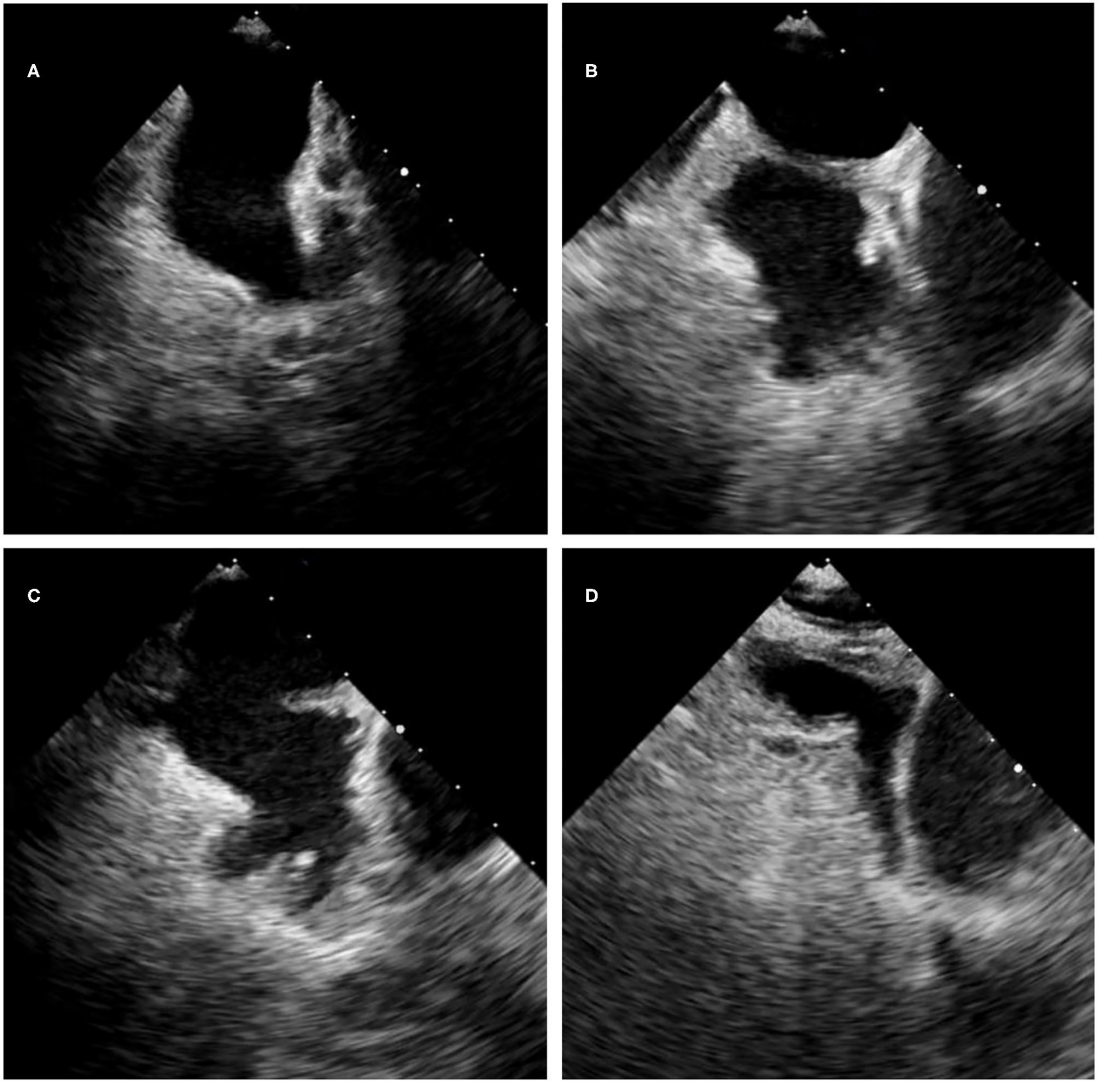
The LAA morphology by 2D transesophageal echocardiography. (A) The chicken-wing morphology. (B) The cauliflower morphology. (C) The cactus morphology. (D) The windsock morphology.
